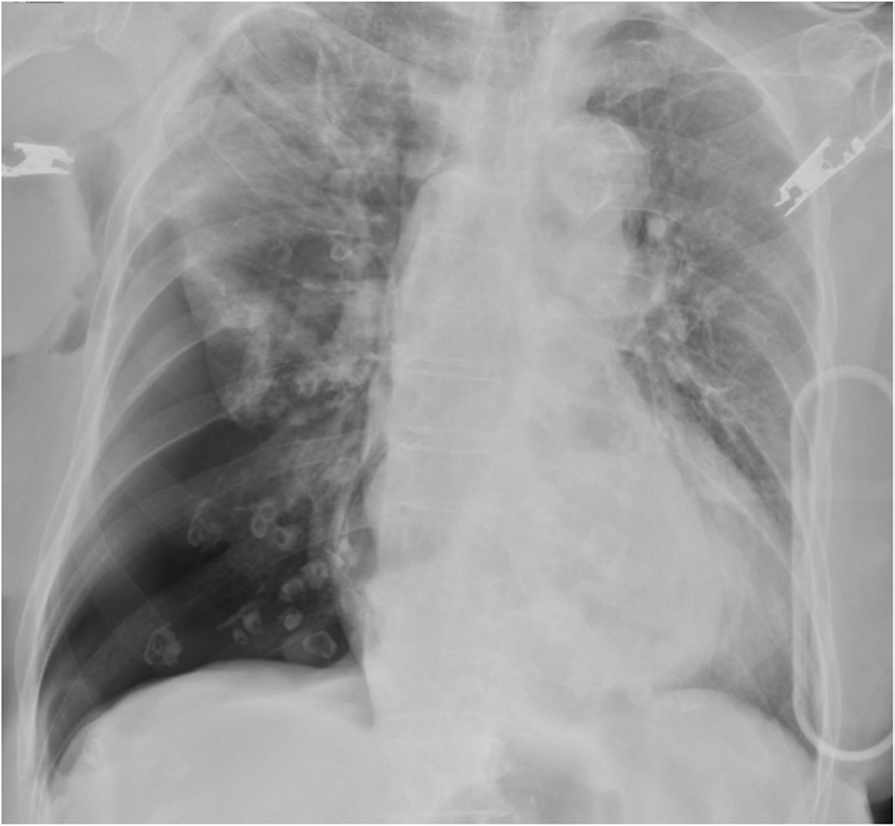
Chest X-ray reveals lung collapse in the right lower lung field, with a possible adhesion of the upper lobe to the chest wall
